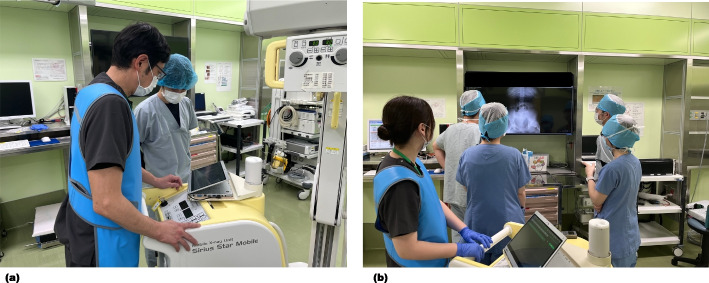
Images of the surgical team checking the radiographs for retained surgical items. a A single surgeon checks the image on a small portable screen to ensure there are no retained surgical items. The radiographer is concerned about whether the imaging area is adequate. b The radiographs are immediately transferred to the electronic medical record and shared on the big screen so that all staff members can view the images together. All members involved in the surgery check for any abnormalities in the images on the big screen. Because the resolution of the images on both screens is the same, an abnormality that is invisible on the small screen may also be invisible when viewing the image on the big screen. However, when in doubt, the images can be rechecked by adjusting the brightness and contrast
